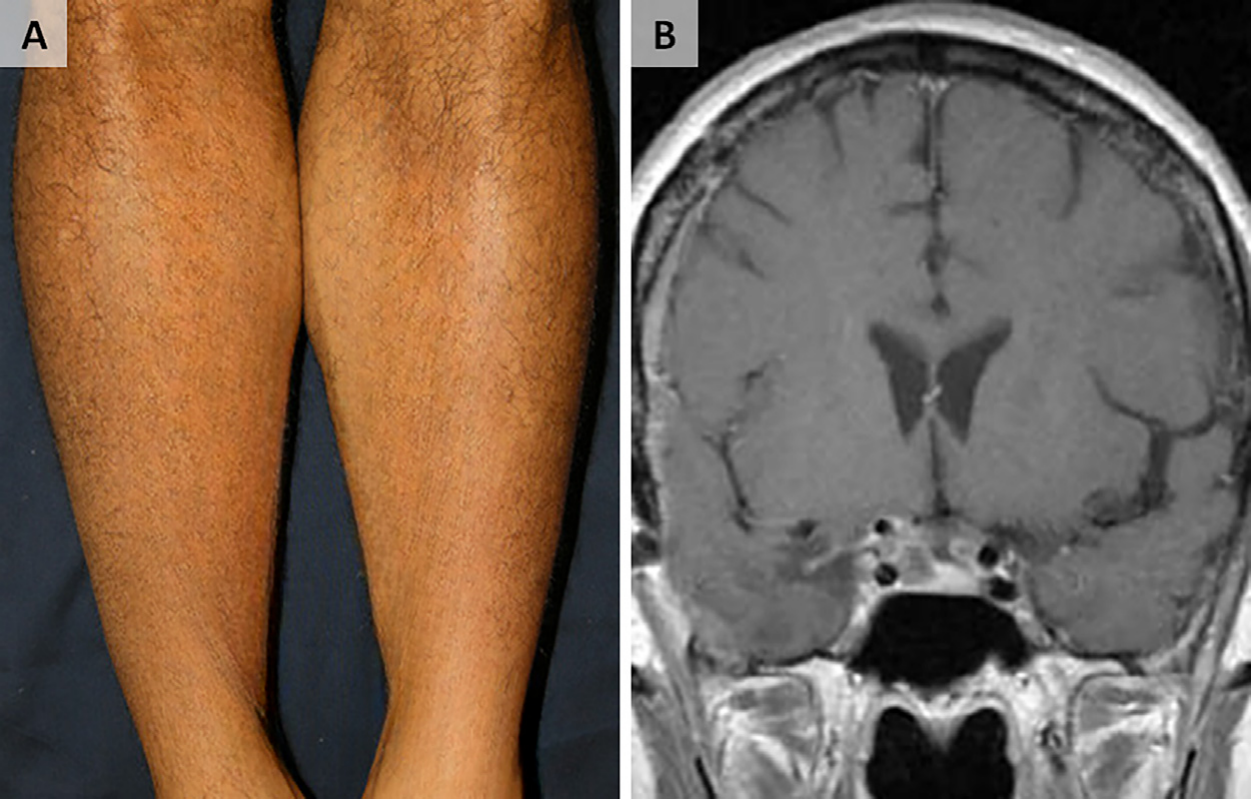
Complete resolution of dermatological and CNS lesions following antimicrobial therapy. (A) Clinical image of the patient's legs, taken 11 months after initiating therapy, showing complete resolution of the previously described papules. (B) Coronal postcontrast T1-weighted MRI sequence, demonstrating complete resolution of the previously noted brain lesions 11 months after starting antimicrobial therapy.
